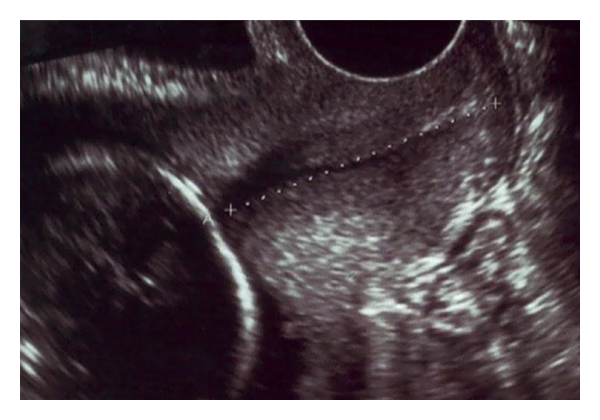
Transvaginal ultrasound image of the uterine cervix.
